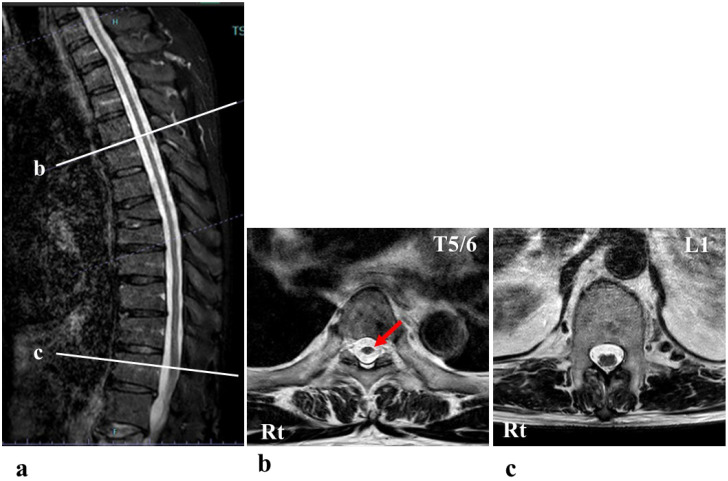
Mid-sagittal section (a) and axial sections at the T5/6 level (b) and L1 level (c) of the T2-weighted magnetic resonance image showed that his thoracic spinal cord was slightly atrophic (arrow in (b)).
